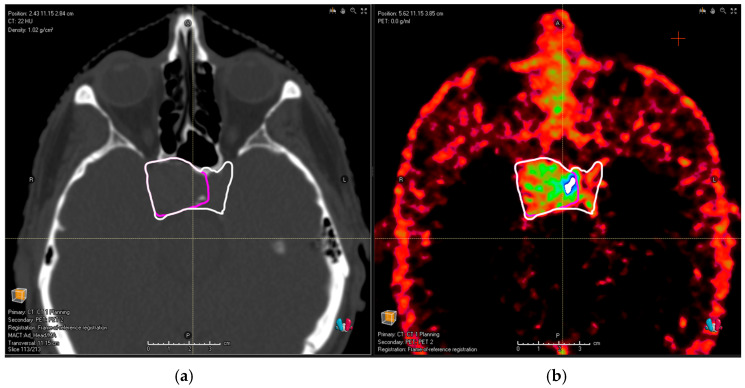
(a) Planning the CT image of a patient with pituitary adenoma. Pink contour shows common MRI volume; after the addition of PET/CT, (b) the observers identified an infiltration in the left cavernous sinus (white contour).
